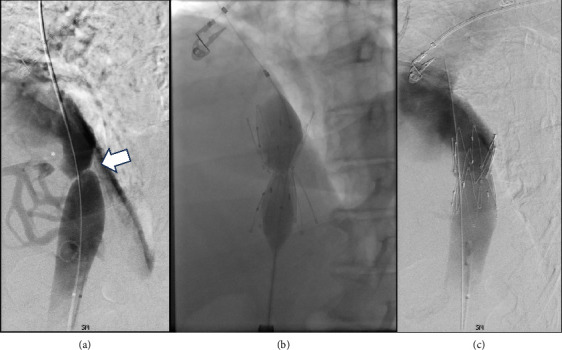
(a) Inferior vena cava venogram demonstrating a narrow web (denoted by the arrow) of the intrahepatic IVC with a translesional pressure gradient of 16 mmHg (above stenosis 8 mmHg, below stenosis 24 mmHg). Multiple hepatic venous collaterals also highlight a potential web/occlusion of the right hepatic vein (denoted by the asterisk). (b) A 30 × 50 mm Gianturco Z stent (Cook Medical, Bloomington, IN) was successfully deployed across the stenosis and subsequently postdilated. (c) Completion venography and intravascular ultrasound demonstrate subtotal luminal restoration with a decreased pressure gradient of 1 mmHg (above prior stenosis 18 mmHg, below prior stenosis 19 mmHg).
